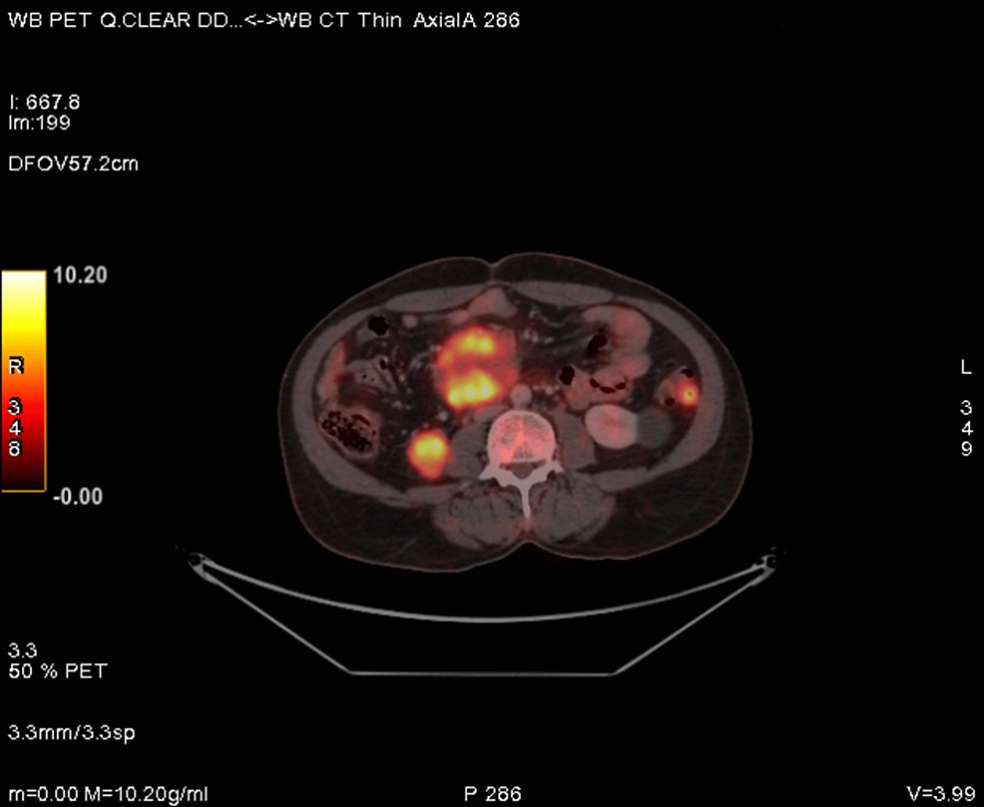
PET-CT showing FDG avid enlarged peri gastric, peri pancreatic, lower para-aortic, mesenteric, and left common iliac lymph nodes. FDG: 18-fluorodeoxyglucose; PET/CT: positron emission tomography/computed tomography
